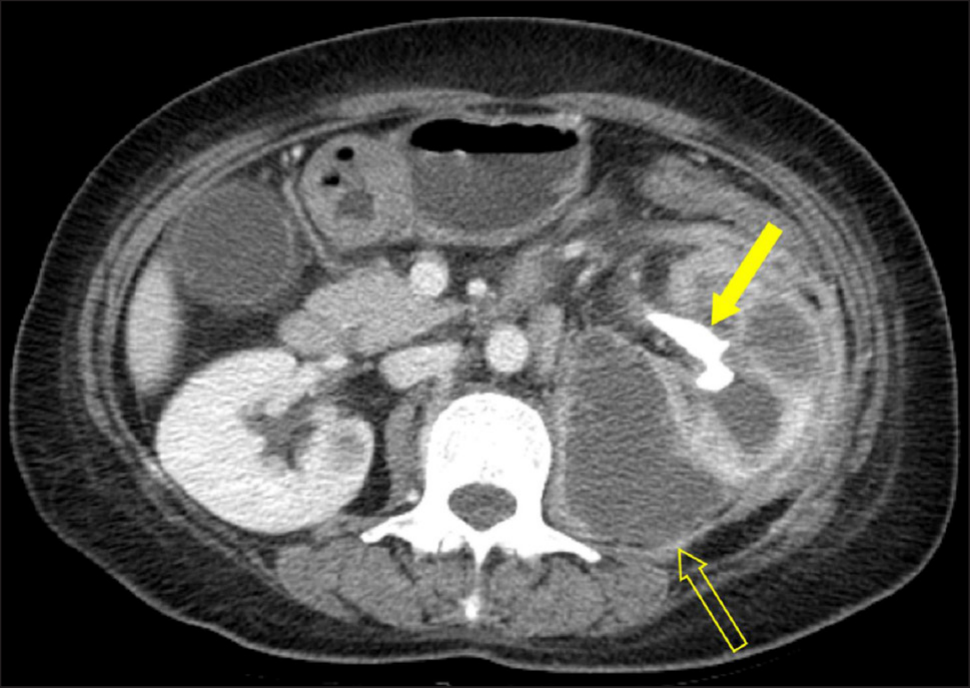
Axial contrast-enhanced CT image shows a calculus (arrow) within the contracted left renal pelvis and perirenal abscess (open arrow) involving the left psoas muscle.
